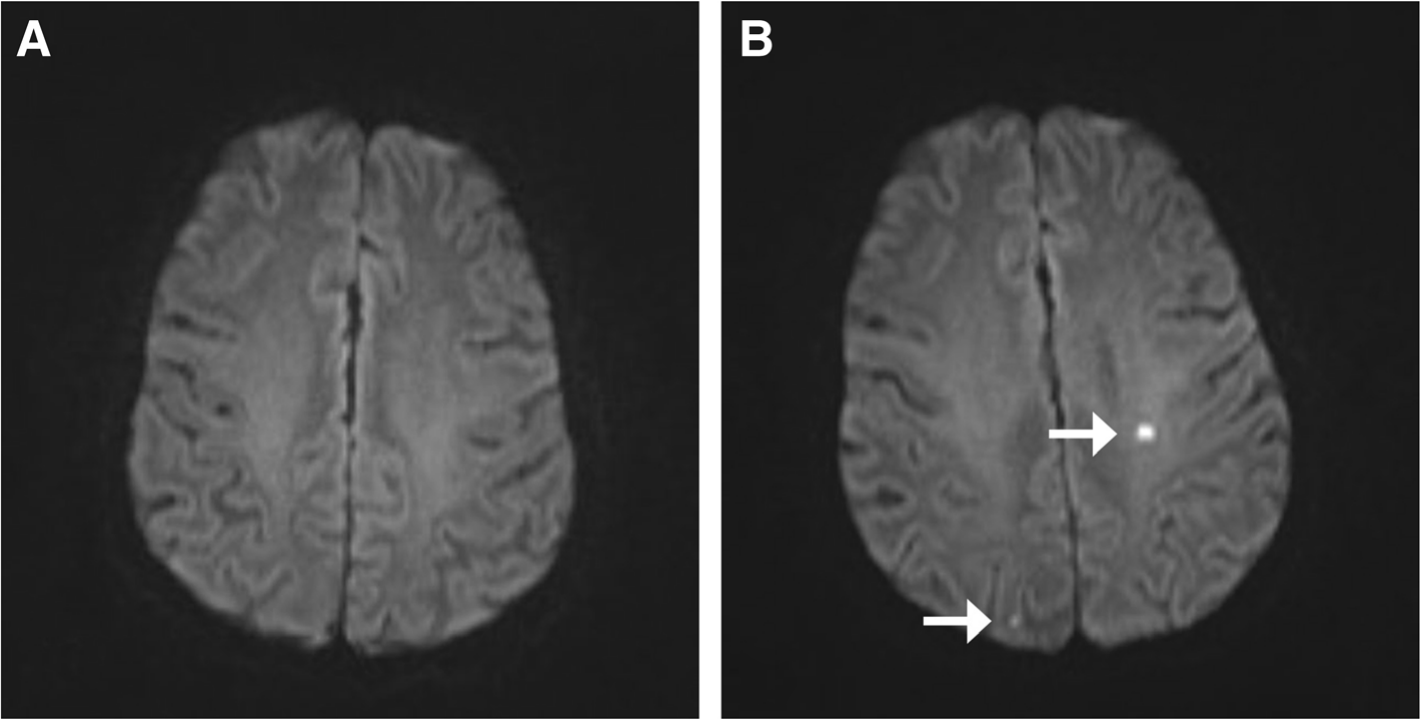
Diffusion-weighted magnetic resonance imaging (DWI) scans of a Perfusion Pressure Cerebral Infarct (PPCI) pilot study participant before (a) and after (b) heart surgery with the use of cardiopulmonary bypass (CPB). The images reveal two silent cerebral infarcts marked by white arrows
